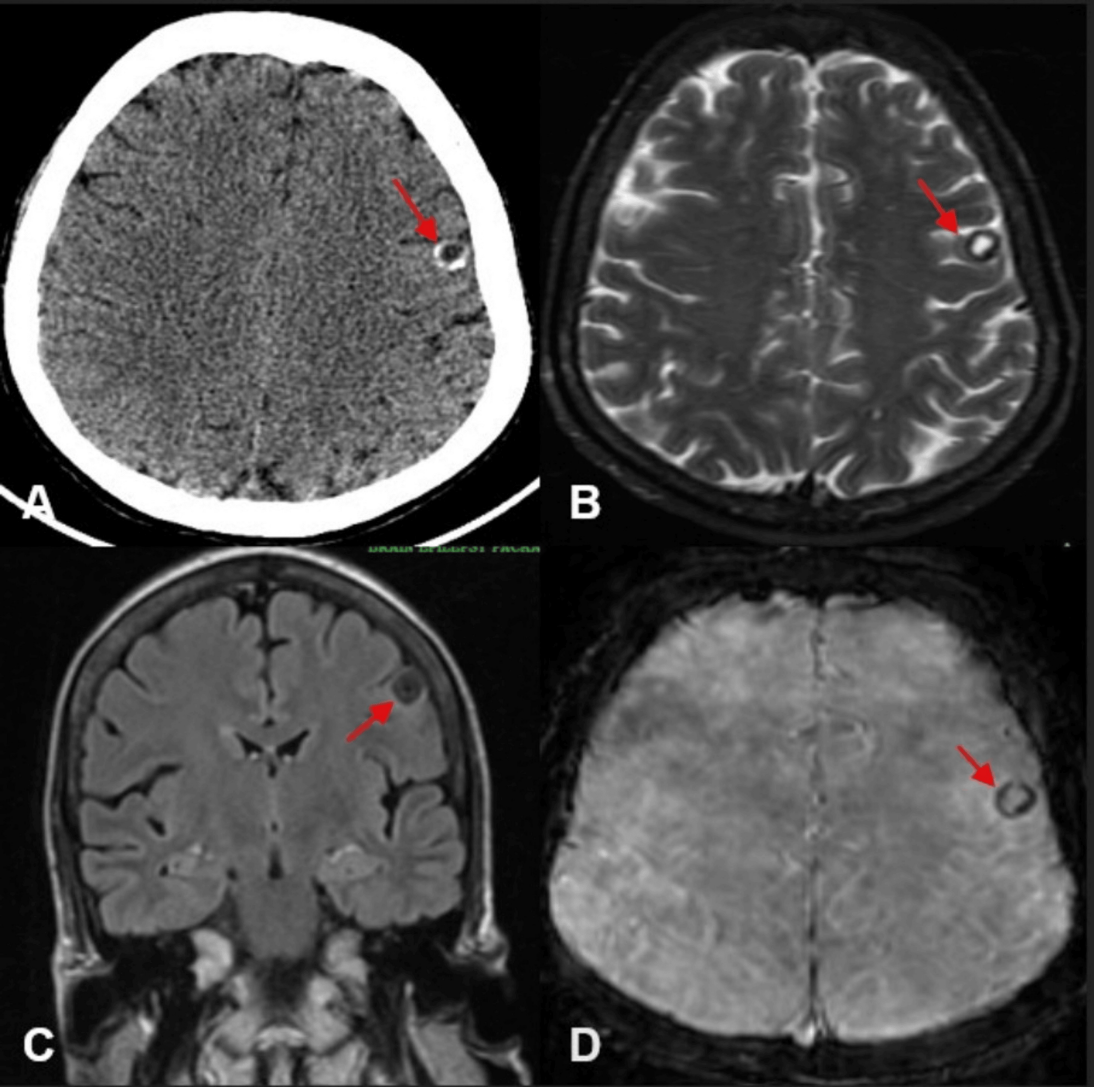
CT and MRI of brain showing neurocysticercosis with wall calcification Axial NCCT image of the brain (A) showing a well-defined hypodense (CSF density) lesion with a peripheral rim of calcification (indicated by red arrow) in the left frontal lobe. MRI brain shows (B) a T2 hyperintense lesion (indicated by a red arrow), (C) FLAIR suppression in the coronal image (indicated by a red arrow), and (D) a peripheral rim of GRE blooming (indicated by a red arrow). NCCT, Non-contrast Computed Tomography; FLAIR, Fluid-Attenuated Inversion Recovery; GRE, Gradient Recalled Echo; MRI, Magnetic Resonance Imaging
